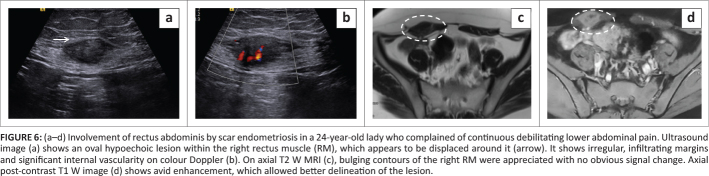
(a–d) Involvement of rectus abdominis by scar endometriosis in a 24-year-old lady who complained of continuous debilitating lower abdominal pain. Ultrasound image (a) shows an oval hypoechoic lesion within the right rectus muscle (RM), which appears to be displaced around it (arrow). It shows irregular, infiltrating margins and significant internal vascularity on colour Doppler (b). On axial T2 W MRI (c), bulging contours of the right RM were appreciated with no obvious signal change. Axial post-contrast T1 W image (d) shows avid enhancement, which allowed better delineation of the lesion.
